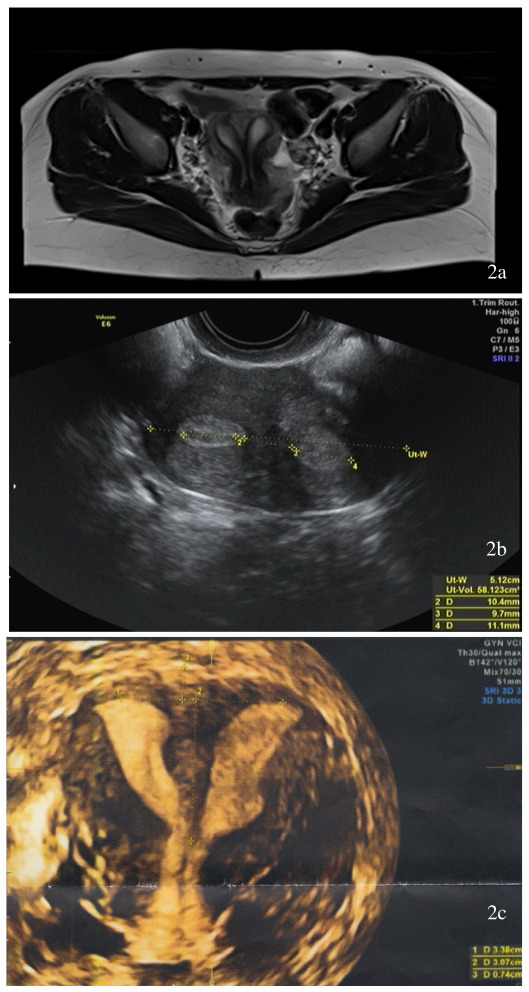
(a) MRI findings before the hysteroscopic resection: complete septate uterus with double “normal” cervix and longitudinal non-obstructing vaginal septum. (b) 2D transvaginal ultrasound. (c) 3D transvaginal ultrasound.
